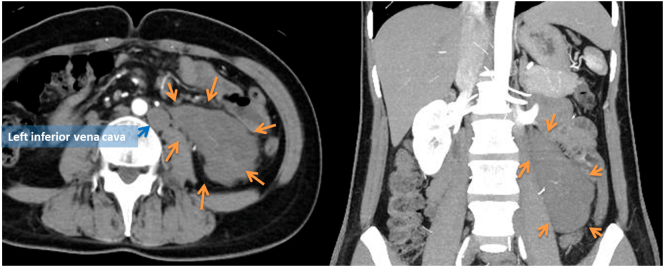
Using contrast-enhanced CT, the tumor was located in the left pararenal cavity to the retroperitoneum. The shape of the tumor was elongated and lobulated with a major axis of 10 cm. This patient had a duplicated inferior vena cava, of which there was no adipose tissue between the left inferior vena cava and the tumor.
